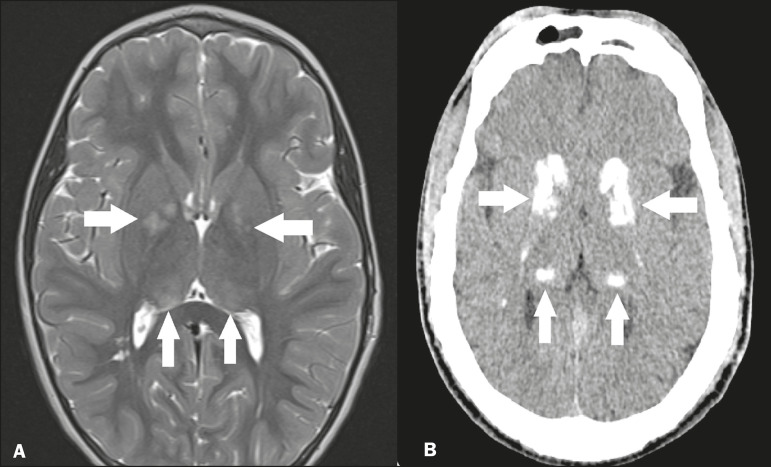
Other toxic-metabolic and degenerative causes of thalamic lesions. A: Axial T2-weighted MRI sequence showing small hyperintense foci in the thalamus and nucleocapsular regions (arrows) in a six-year-old patient with neurofibromatosis type 1 (image courtesy of Dr. Márcio Tadeu Vieira de Brito). B: Axial CT slice showing calcifications in the thalamus and nucleocapsular regions (arrows) in a patient with Fahr’s disease who presented with progressive psychosis.
